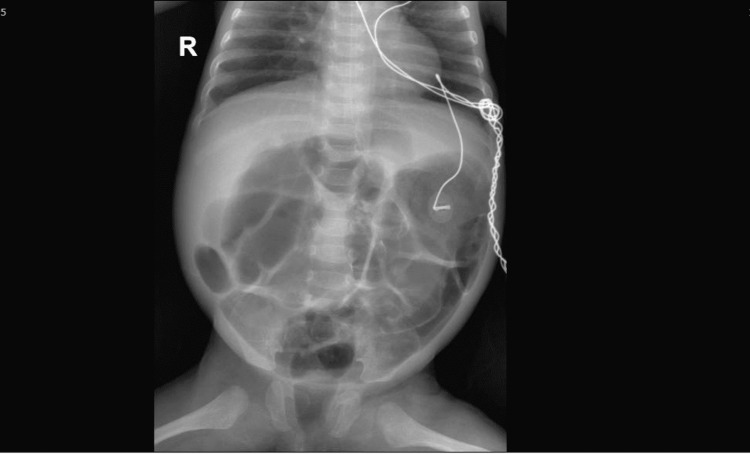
Abdominal X-ray showing abdominal distention
